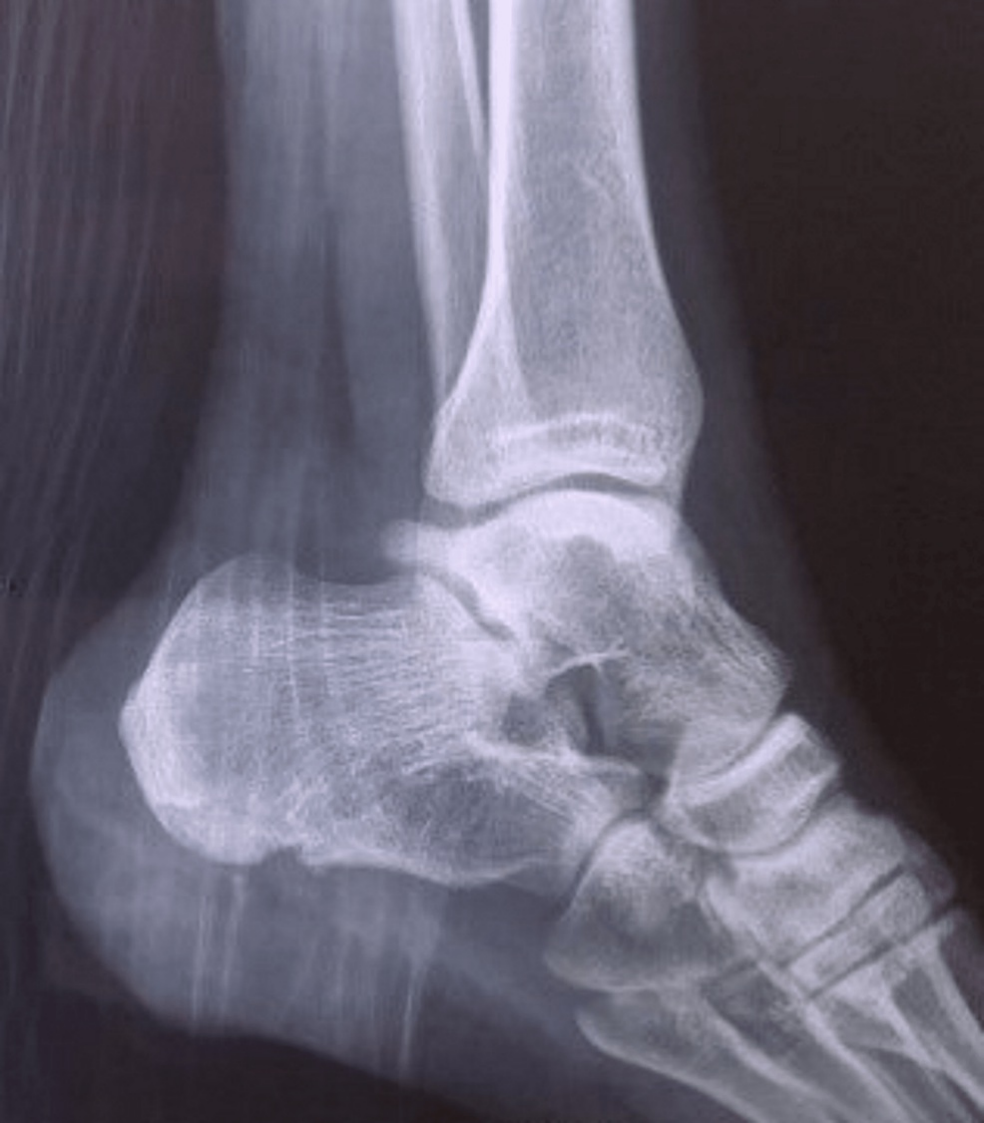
The patient's three-year postoperative follow-up X-ray
